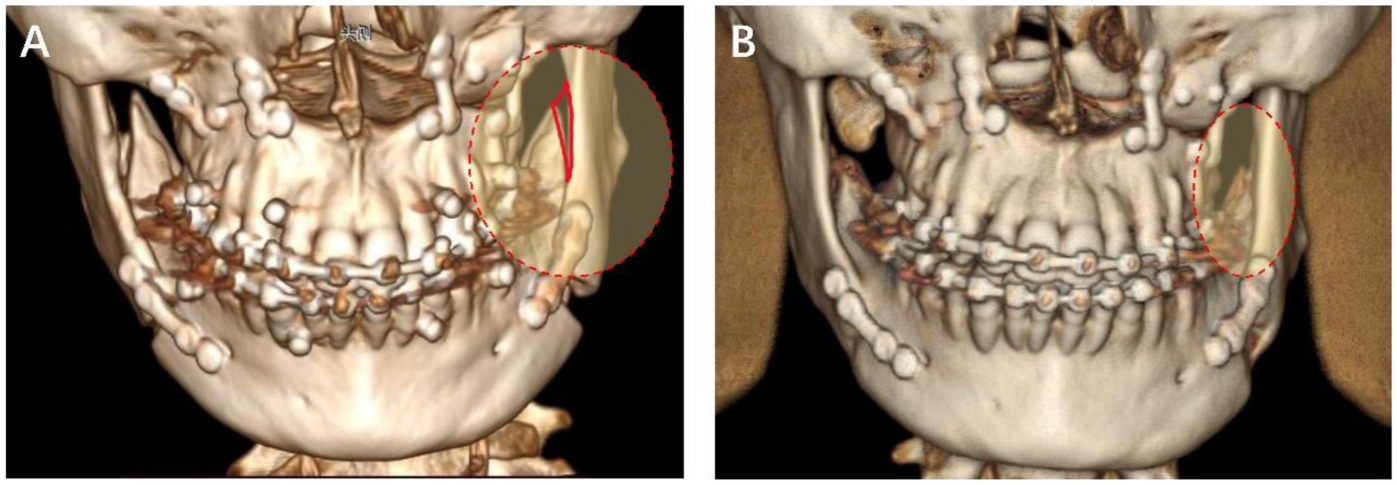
3D reconstruction of CT images post orthognathic surgery. (A) 3 days after surgery. The bony interference in red region was completely removed and the proximal and distal segments were aligned passively. (B) 4 months after surgery. Bone regeneration could be obviously observed in the red region.
